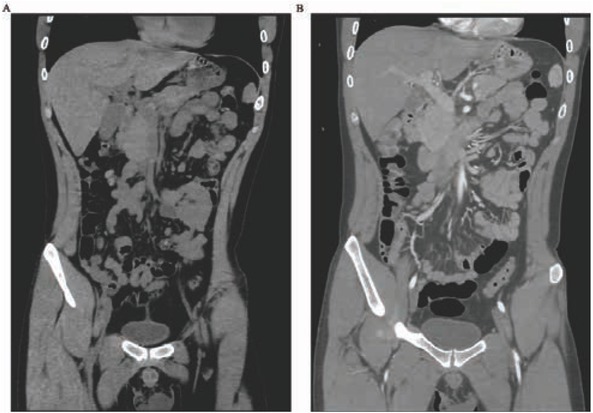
No lesion was found in the Contrast-enhanced CT and the appendix displayed normal.
